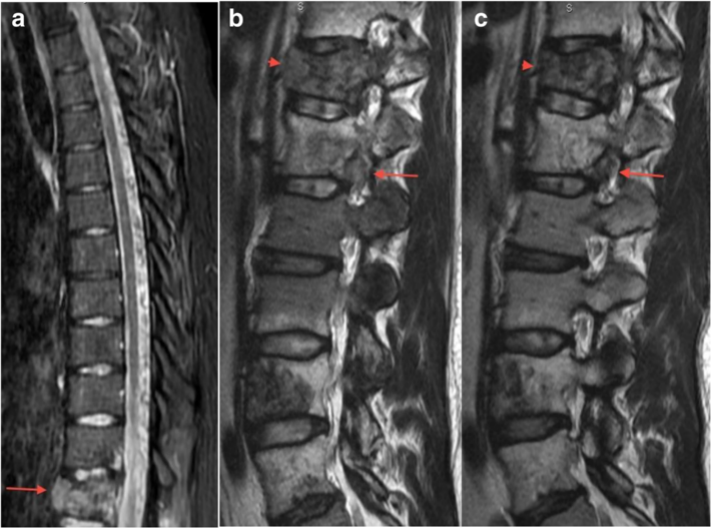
Sagittal MR images of thoracolumbar spine: a pre-treatment STIR demonstrates the lesion at T12 with extension through the anterior vertebral body bony margin; b pre-treatment T2 demonstrates tumour projecting into the T12 prevertebral soft tissue (*arrowhead*) and into the neural foramen at L1 (*long arrow*); and c post 3 cycles of pembrolizumab, there is no longer prevertebral extension of tumour at T12 (*arrowhead*) and only ill-defined soft tissue remains around the L1 root (*long arrow*); lesions in the body of L1, L4 and L5 are also smaller
